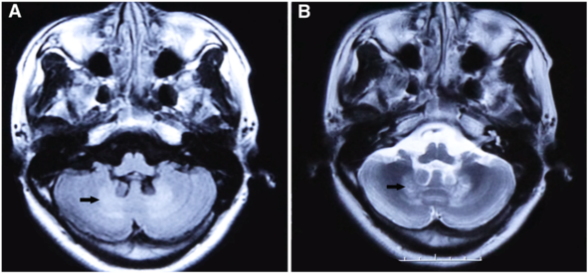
MRI results: brain. Brain MRI shows T1-weighted (A, arrow) and T2-weighted (B, arrow) hyperintensities in the dentate nuclei.
